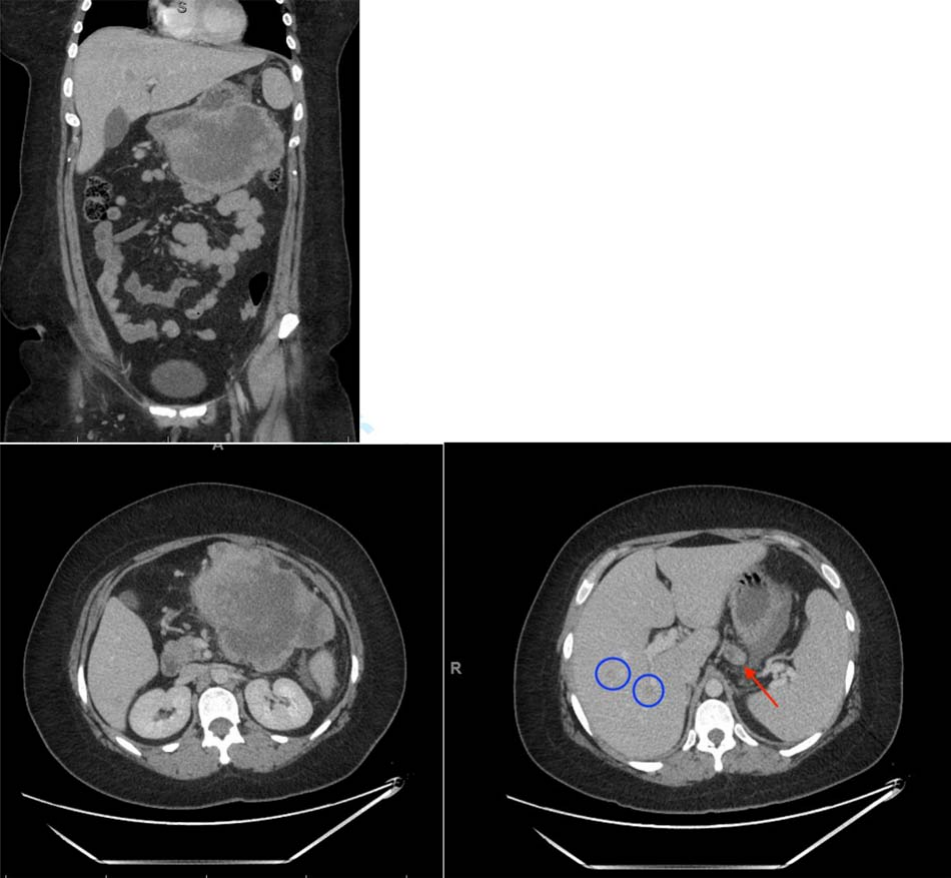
Initial CT scan showing large epigastric mass originating from the greater curvature of the stomach. Red arrow indicates large necrotic lymph node. Blue circles indicate liver metastases.
